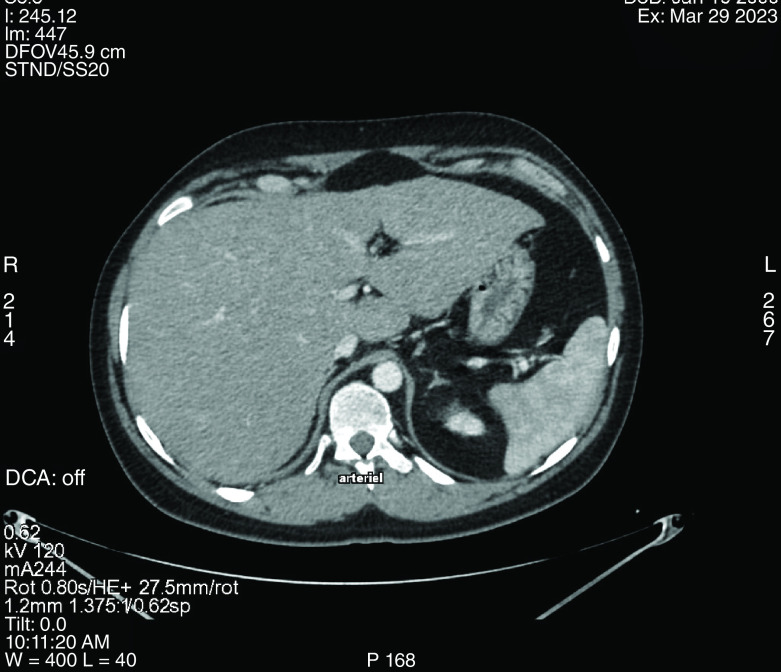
Abdominal CT scan showing a diffuse nodular hepatic infiltration.
